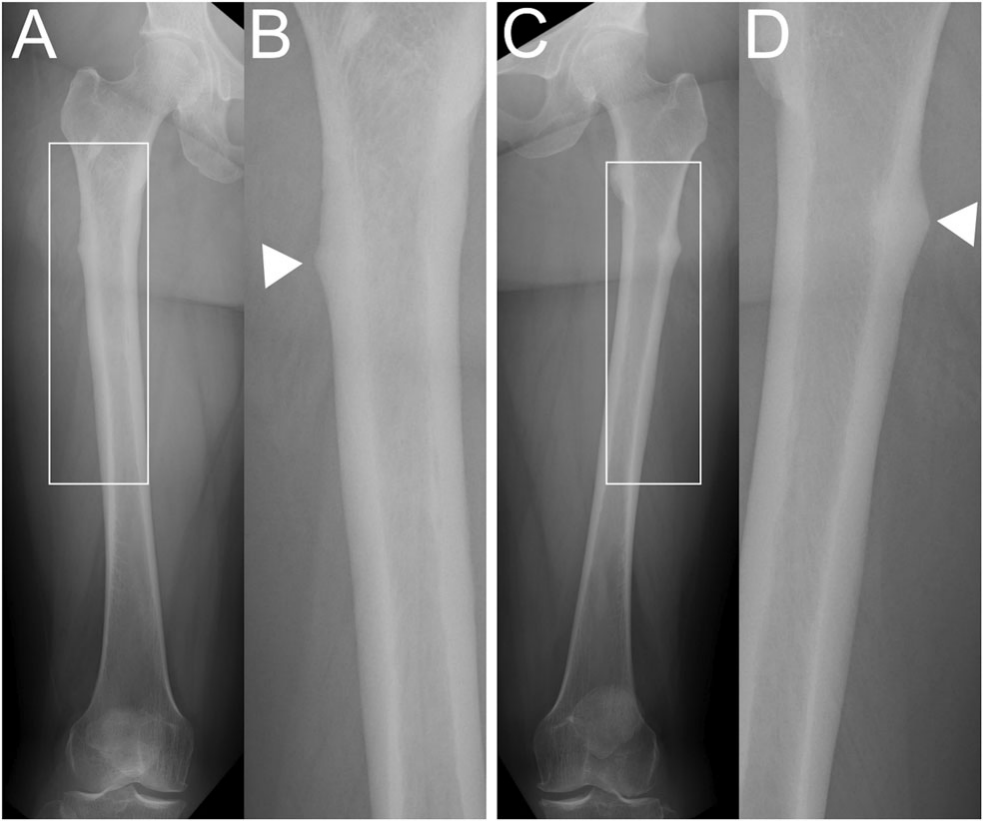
Images for patient with grade 2b radiographic findings. The patient was a 52‐year‐old woman with bone metastasis from breast cancer who had received oncologic denosumab for 74 months. (A) Anteroposterior image of right femur. (B) Enlarged image of white box in Fig 3A showing apparent local thickening of subtrochanteric lateral cortex (arrowhead, TR 1.5). The thickening had no radiolucent fracture line. (C) Anteroposterior image of left femur. (D) Enlarged image of white box in Fig 3C showing apparent local thickening of subtrochanteric lateral cortex (arrowhead, TR 1.9). The thickening had no radiolucent fracture line. Patient was assessed as grade 2b. TR, thickening ratio.
